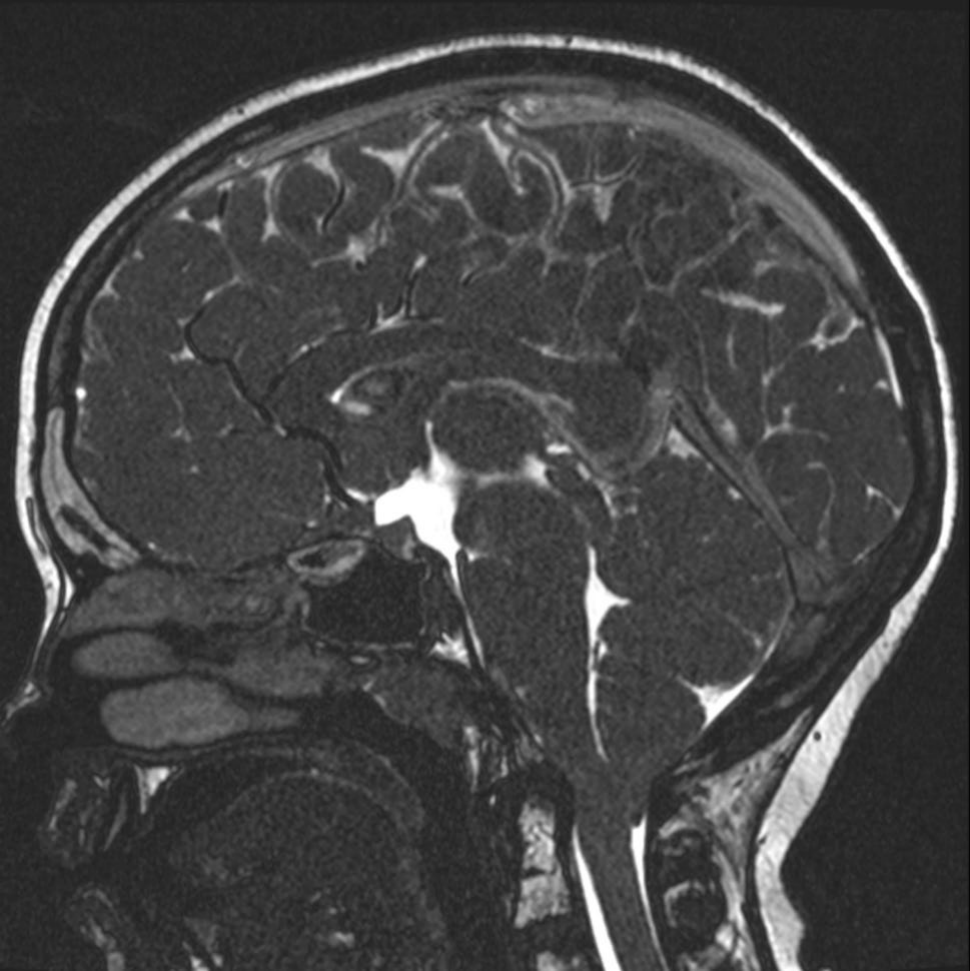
MRI demonstrating cerebellar sagging at the time of clinical presentation
